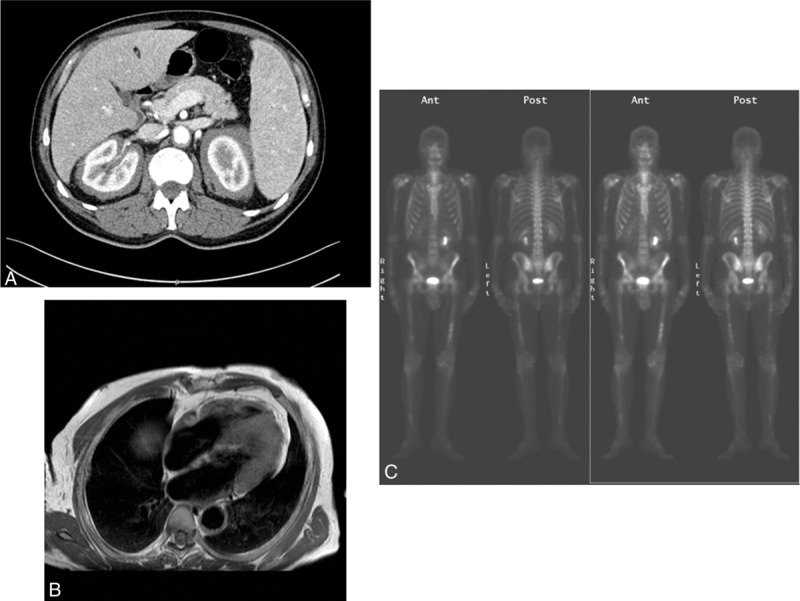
A, Contrast-enhanced abdominal CT scan showing solid vascularized tissue located in the retroperitoneal space surrounding the kidneys, ureters and aorta. B, Cardiac MRI showing a mass located in the right atrioventricular sulcus. C, Bone scan showing symmetrically irregular radiotracer uptake in the long bones of the limbs.
